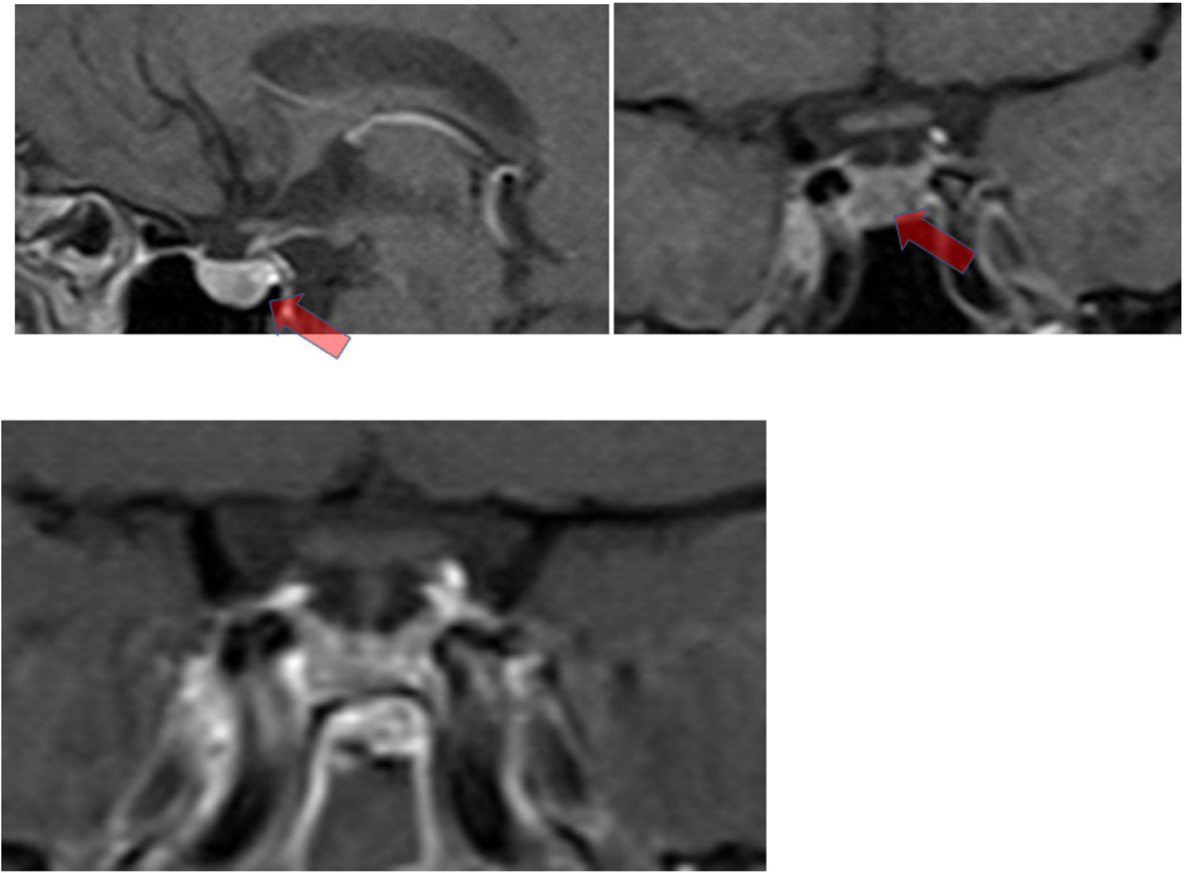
Pituitary magnetic resonance imaging. Arrow: The most hypocaptizing (darkest gray) region, with rounded aspect, in the right half of the pituitary gland, passing the midline to the opposite side and prophesying to the sphenoid sinus in relation to the pituitary adenoma
